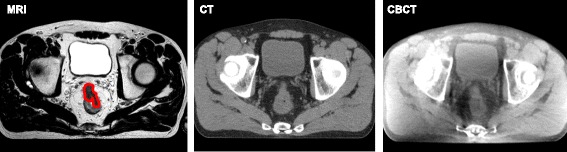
Transverse images of the lower abdomen of a rectal cancer patient using different imaging modalities. The images are of the same patient, depicting the same location through the rectal tumor. On the left; MRI, in the middle; computed tomography (CT) and at the right; CBCT. The tumor is delineated in red on the MR image. Note: on CT and CBCT images, there is contrast between the rectum and surrounding mesorectal fat, but no contrast between the rectum and the tumor
